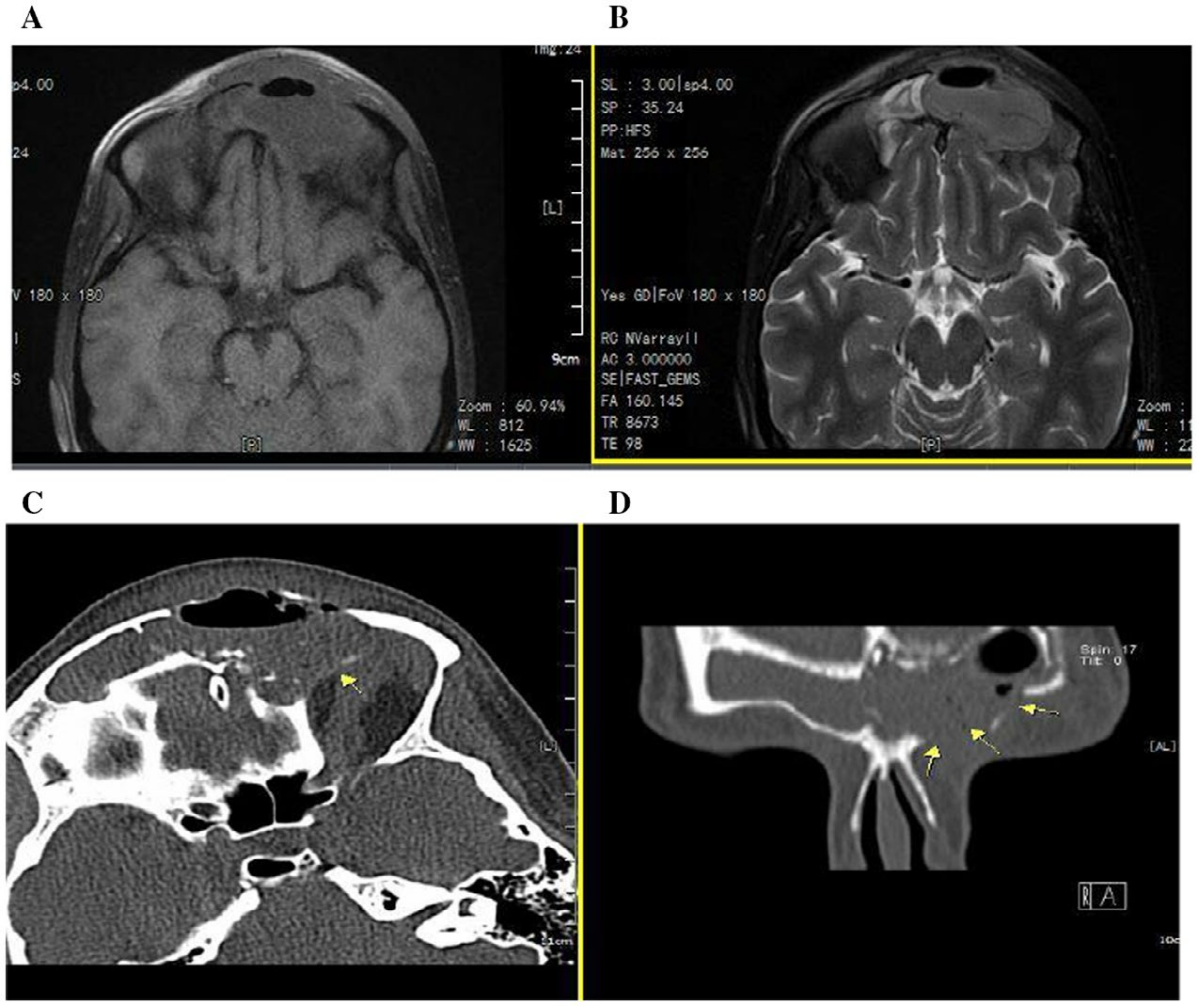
Brain MRI. (A and B) In MRI images, para nasal sinuses were depicted expansion and opacification of the left frontal sinus. (C and D) The anterior and posterior sinus wall have bowing toward the left orbit and frontal scalp associated with disruption (yellow arrows) of bony sinus walls and inflammatory changes in the left frontal scalp.
